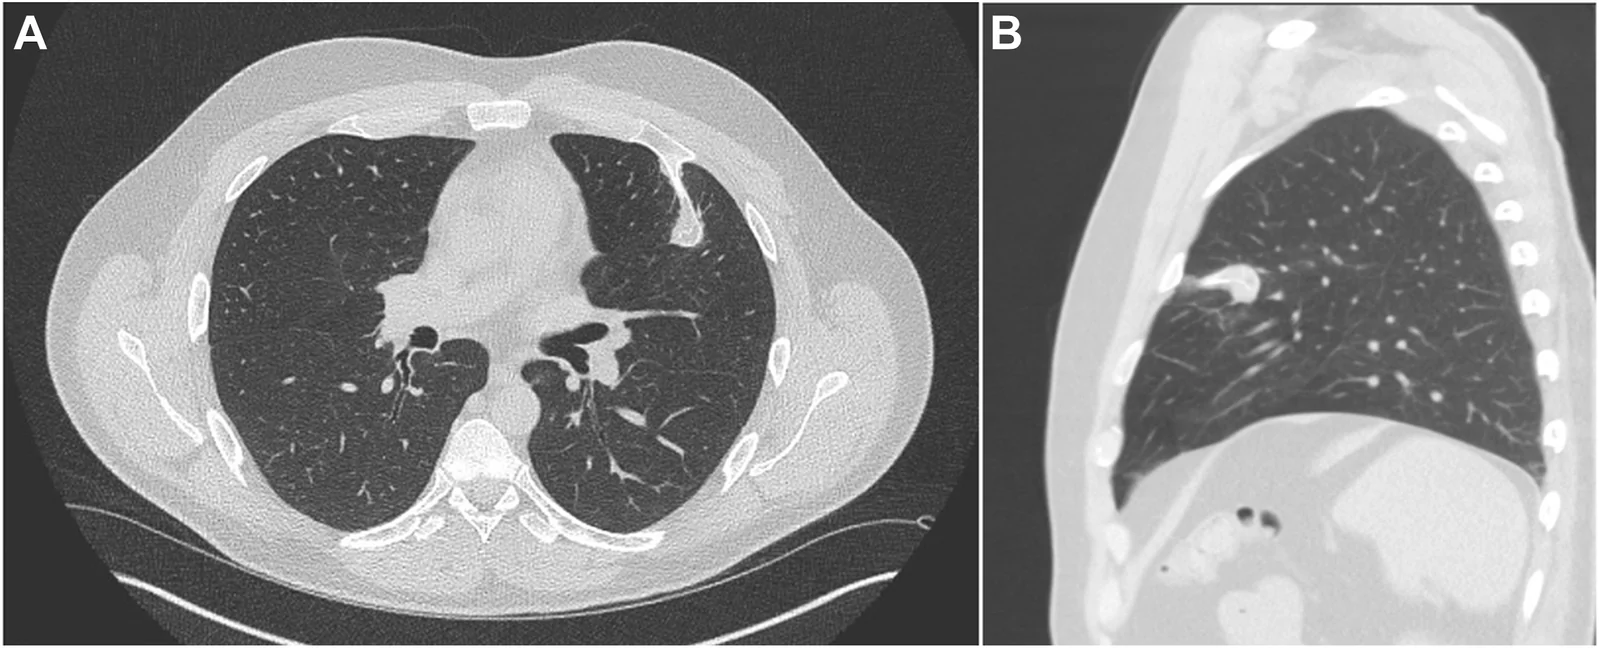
Computed tomography scan of lesion. (A) Axial view of anterior left third rib elongated lesion extending into intrathoracic cavity/lung. (B) Sagittal view of anterior left third rib lesion.
